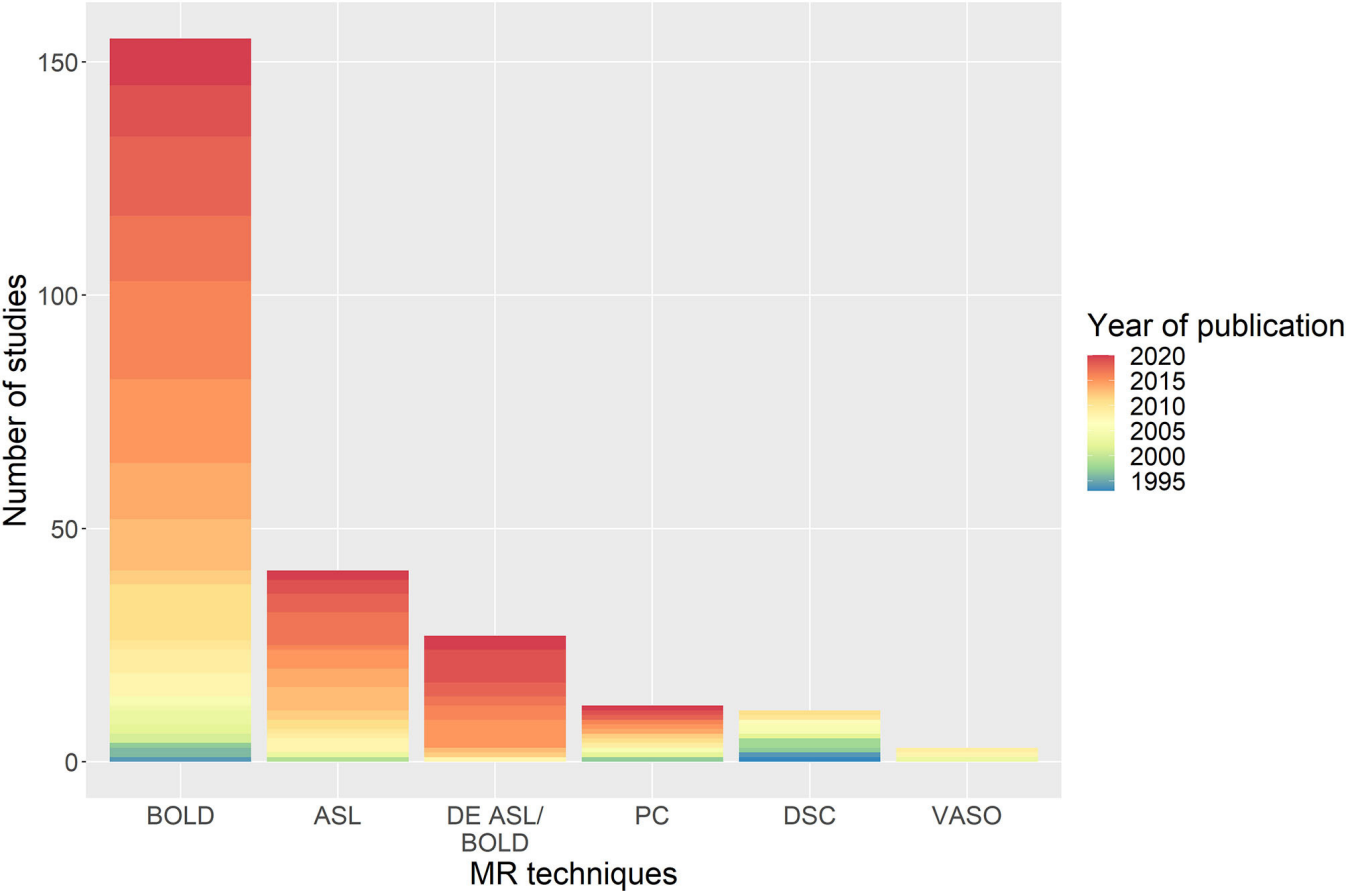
Distribution of the MRI sequences used in studies with the associated year of publication of the paper. BOLD, blood-oxygen-level-dependent; ASL, arterial spin-labelling; DE, dual-echo; PC, phase-contrast; DSC, dynamic susceptibility contrast; VASO, vascular space occupancy.
